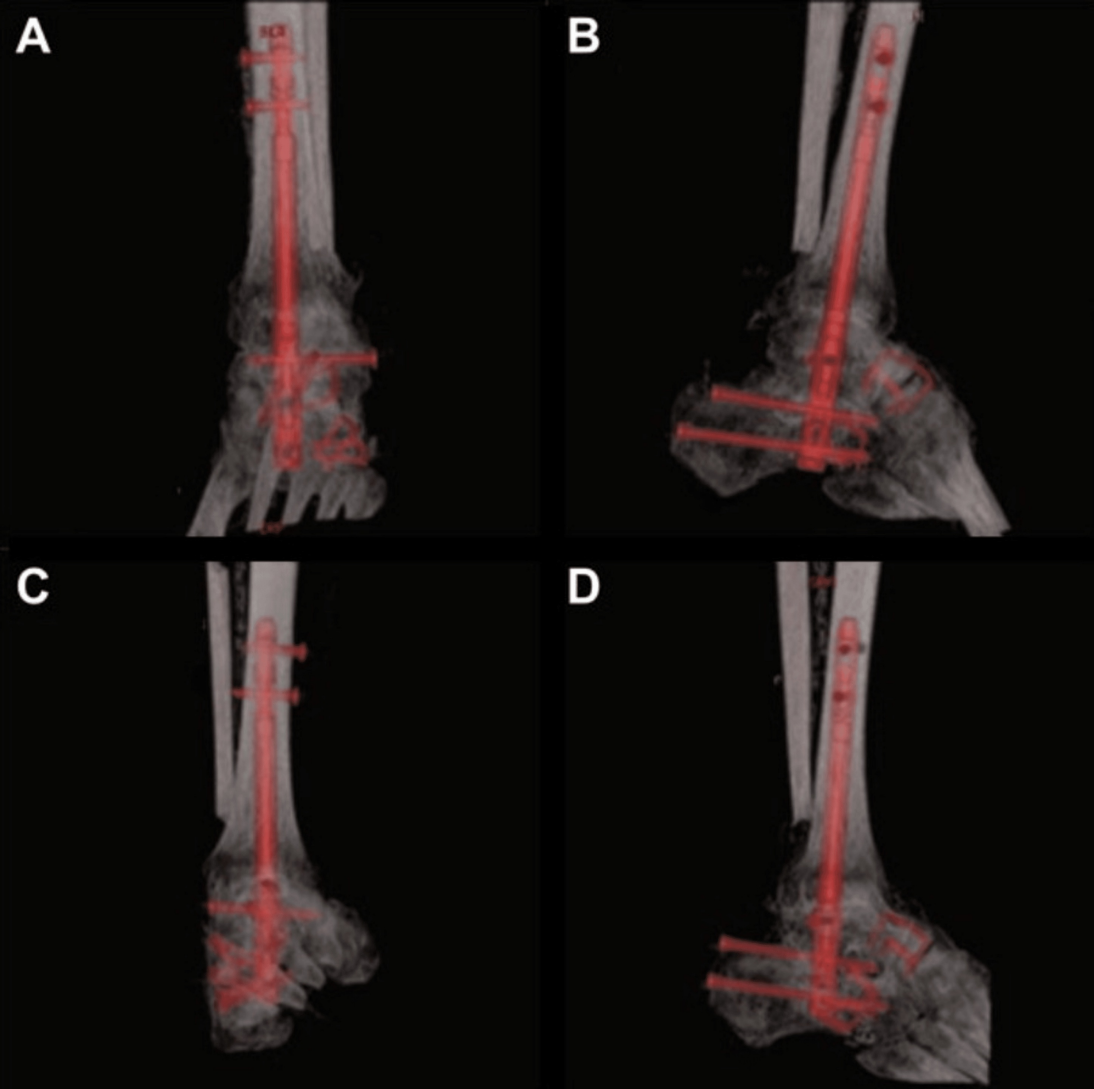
Nine-month postoperative CT scans of bilateral feet A: Coronal view of the left ankle, B: sagittal view of the left ankle, C: coronal view of the right ankle, D: sagittal view of the right ankle The intramedullary nail appears in red on all views to enhance visualization. Osseous fusion is noted across the tibiotalar and subtalar joints bilaterally with maintained alignment and no evidence of hardware complications. CT: computed tomography
